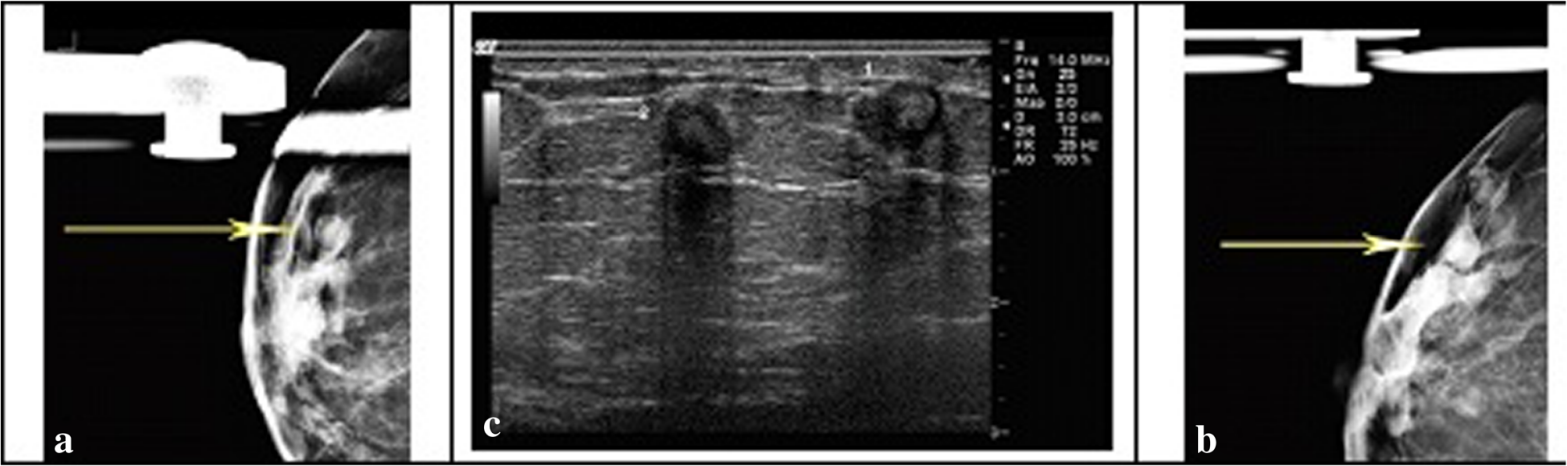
Craniocaudal mammograms and right breast mediolateral oblique. a and b) show round masses with radiolucent centers at the site of palpable finding. c) Ultrasound of the right breast at site of palpable finding demonstrate two hypoechoic round masses with central echogenicity with associated posterior acoustic shadowing (Derived in accordance with William et al. [19])
